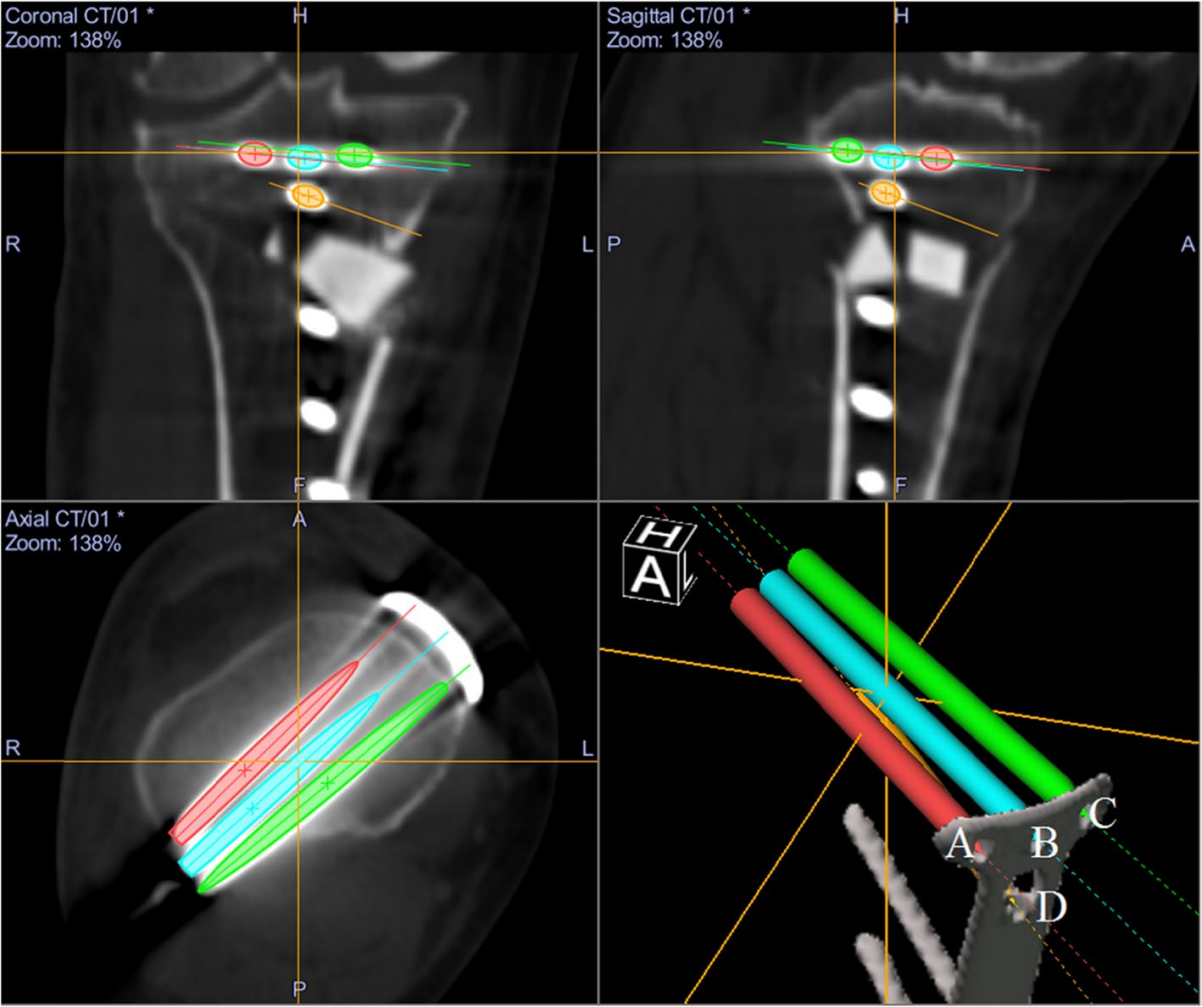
To avoid halation of locking screws on the CT image, another cylinder was made so that the centre of the cylinder passed the centre of the screw head and the tip of the screw. Four locking screws for the proximal tibial fragment were named A–D screws (A Proximal anterior screw; B Proximal middle; C Proximal posterior; D Distal)
